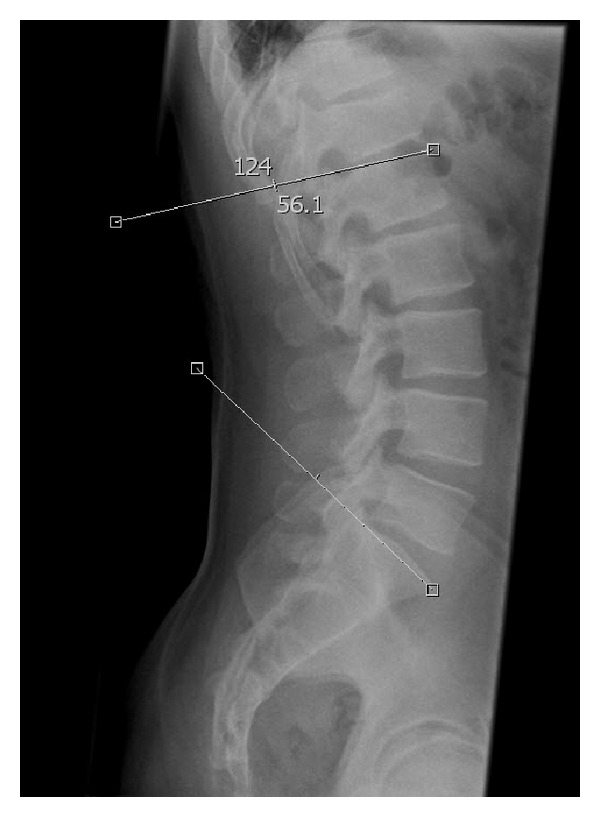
Anatomical Cobb angle measurement in conventional radiograph.
